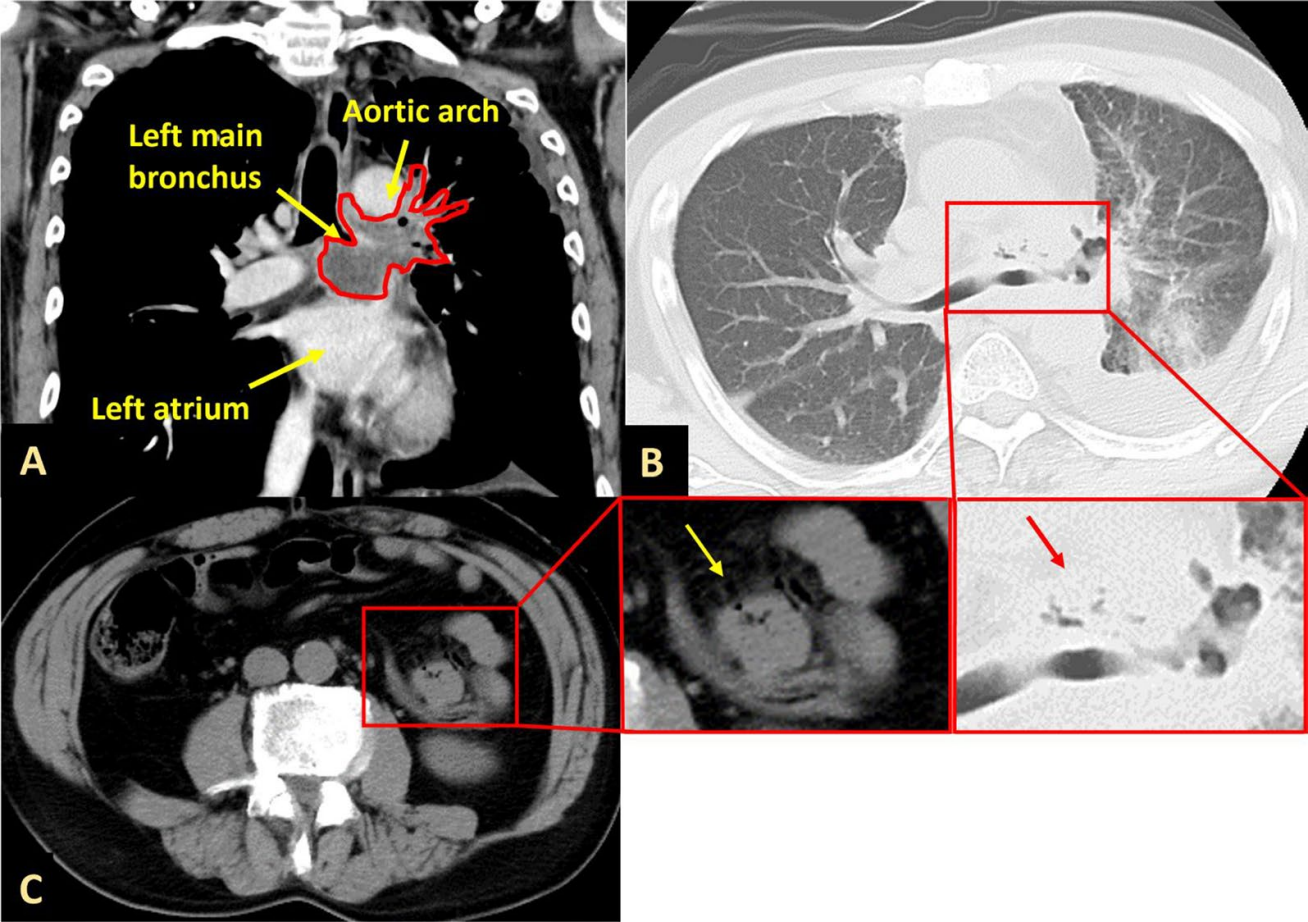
Chest computed tomography (CT) image showing a left pulmonary hilar mass invading the left atrium and the left main bronchus (A) and air densities (arrow) inside the tumor (B). Abdominal CT image showing air densities (arrow) around the intestinal membrane (C). Window level/window width: A, C: (40/300), B: (−700/1800)
